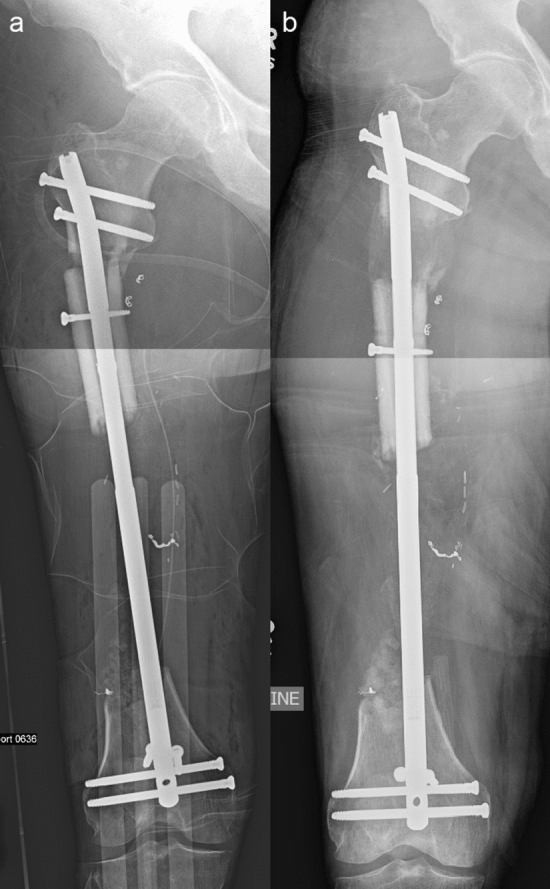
Anteroposterior radiographs of the right femur of a Bone Transport Nail prior to imaging with a 3.0 T MRI (a). Forty-five days after the imaging was completed, anteroposterior radiographs demonstrate that the nail was still able to be activated and continue bone transport (b)
